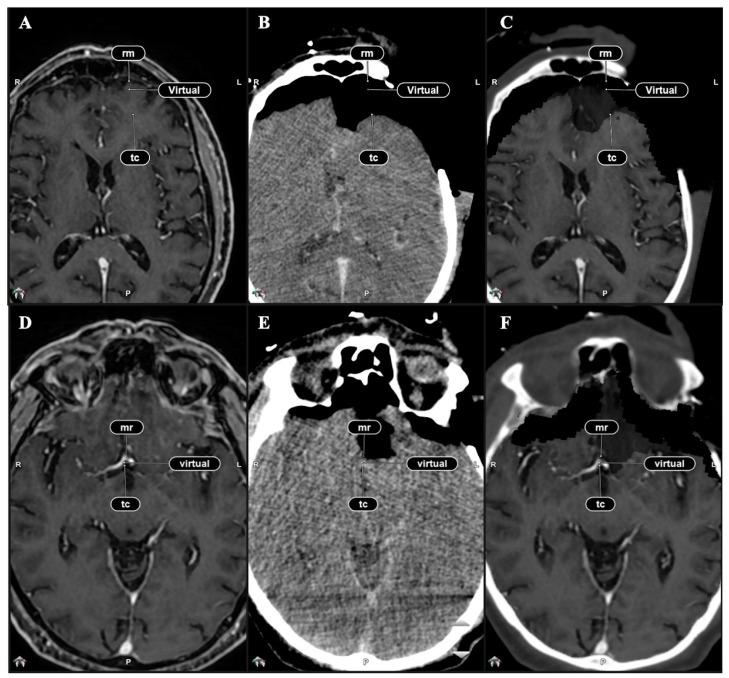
Identification of corresponding anatomic landmarks used for TRE calculation after RIF and EIF. (A) Pre-operative MRI with corresponding cortical points. (B) iCT scan image with corresponding cortical point. (C) Virtual iMRI with corresponding cortical points. (D) Pre-operative MRI with AComm set as reference point. (E) iCT scan image with corresponding AComm. (F) Virtual iMRI with corresponding AComm.
